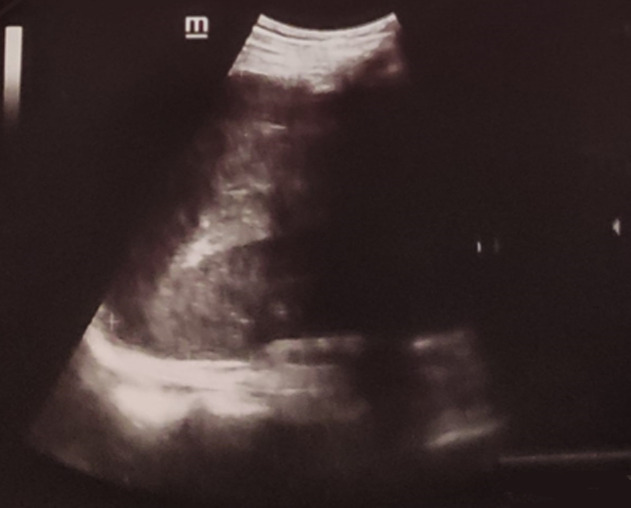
ultrasound showing a hypoechogenic range of the splenic compartment
